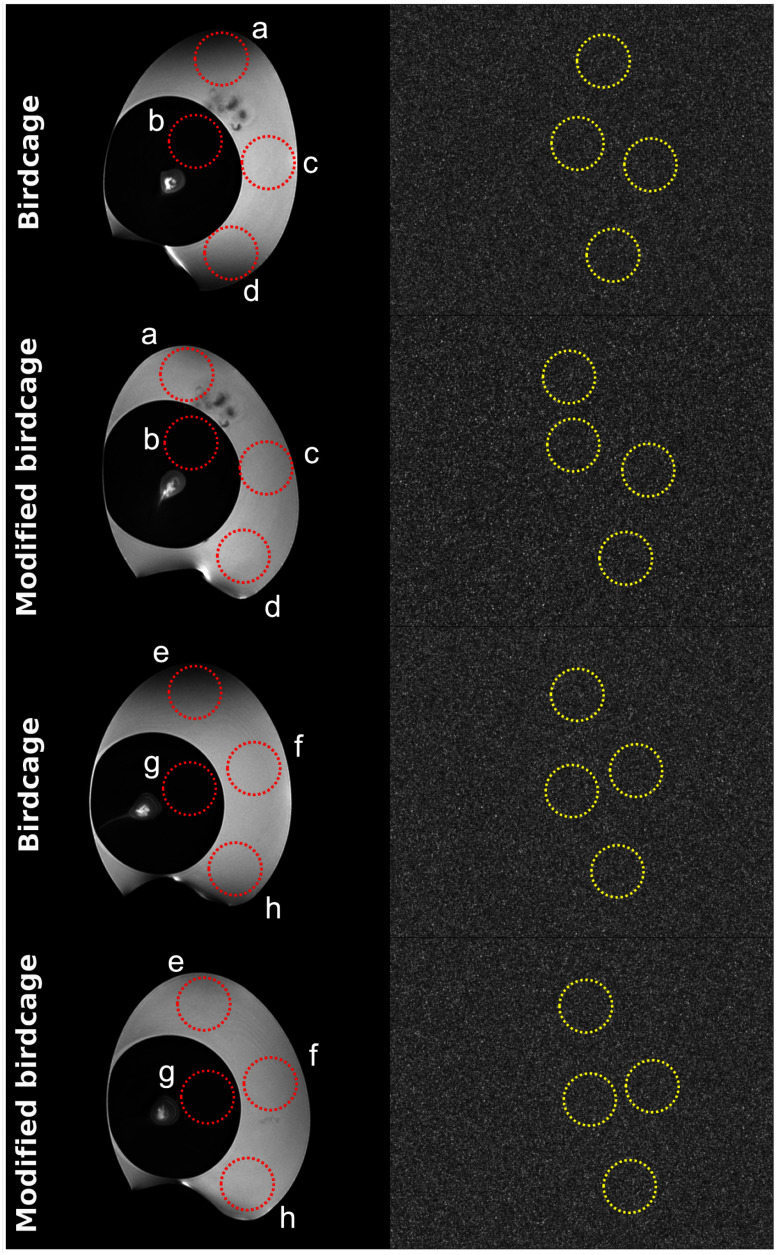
The MR images of supermarket eggs acquired using both birdcage coils, with corresponding noise images displayed on the right. The red and yellow dotted circles within the images represent various selected ROIs and the values in these regions are used for the calculation of SNRs.
